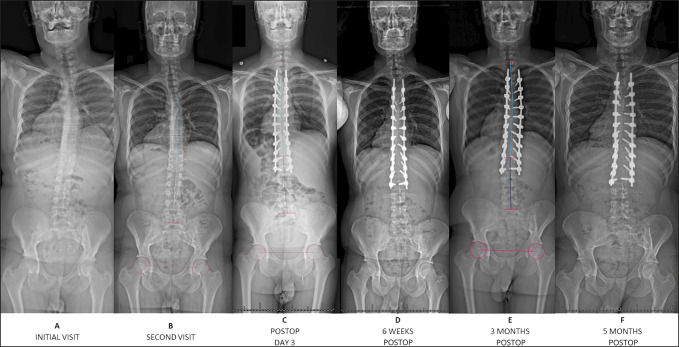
Standing anterior-posterior radiographs from the initial visit to 5 months postoperatively. A and B, Preoperatively, the patient had severe thoracic kyphosis. C, Radiographs show posterior-only multilevel Smith-Peterson osteotomies from T7 to T11 and pedicle screw instrumentation and fusion from T4 to L2. D–F: Postoperative radiographs obtained at 6 weeks, 3 months, and 5 months.
